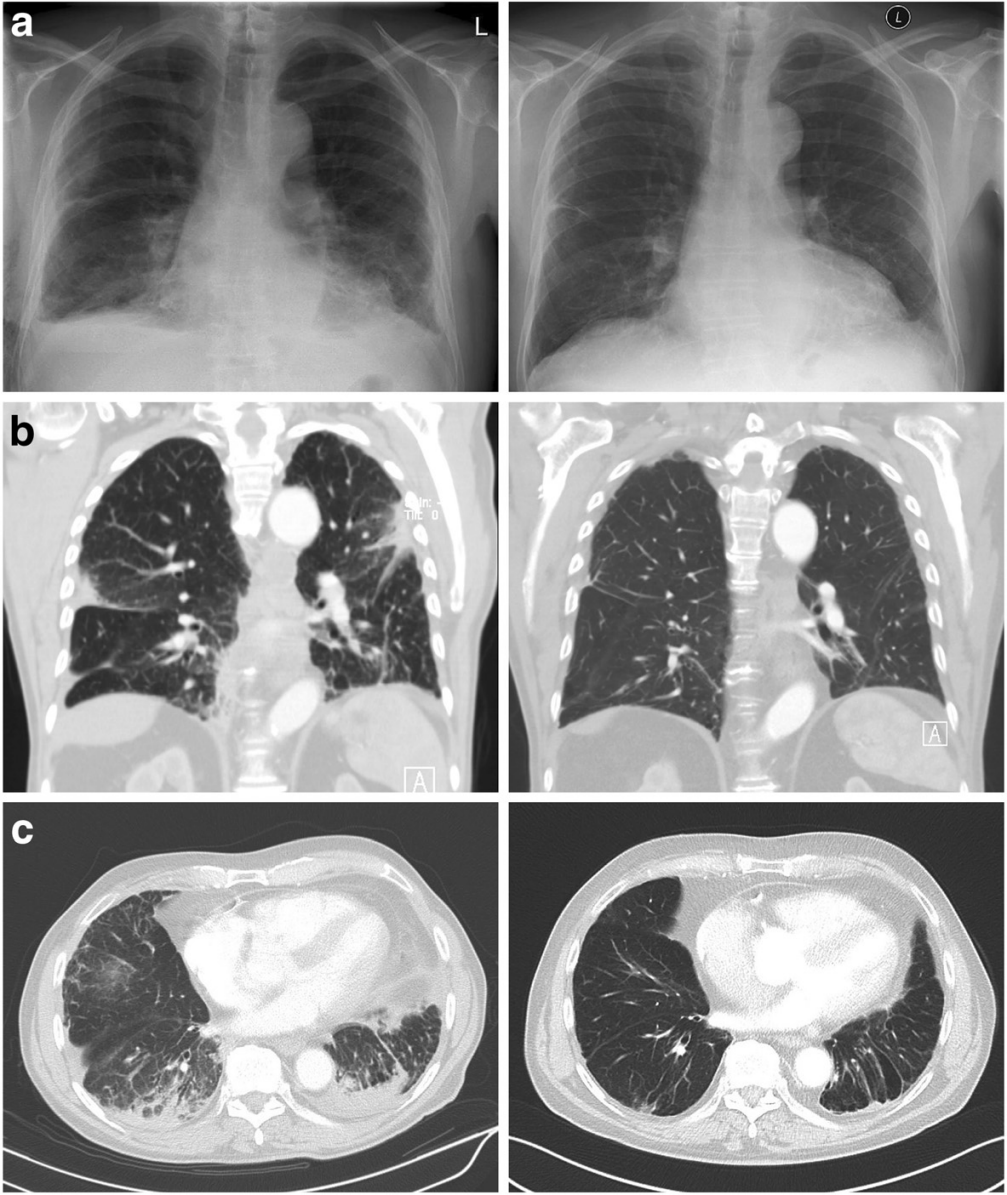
a: *Left*: Chest radiograph obtained upon admission showed inhomogeneous shadows in the lower lobes, right sided pleural effusion and a pleural consolidation in the right middle field.*Right*: significant improvement after 1 month of steroid therapy. b and c: *Left*: CT-scan before treatment start showed bilateral pleural consolidations especially of the lower lobes with air bronchograms and traction bronchiectasis. *Right*: significant improvement after 4 months of steroid therapy.
